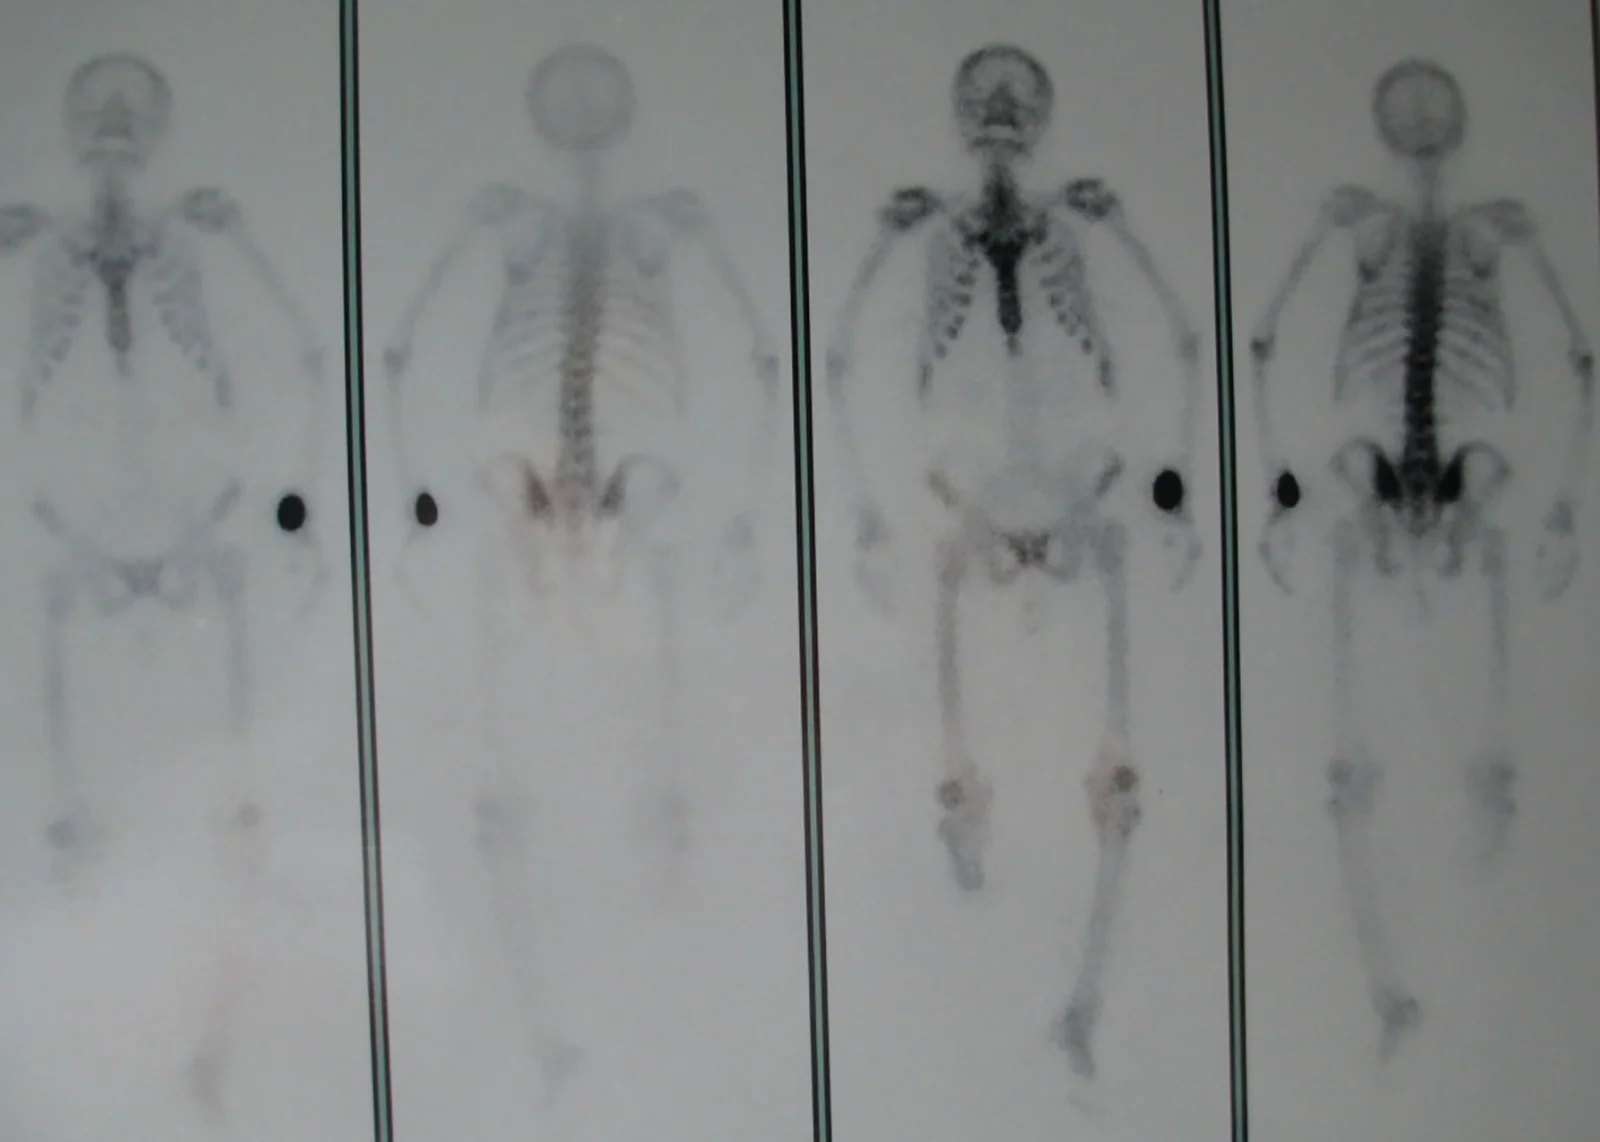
Postoperative bone ECT revealed no evidence of osseous metastasis. ECT = emission computed tomography.
